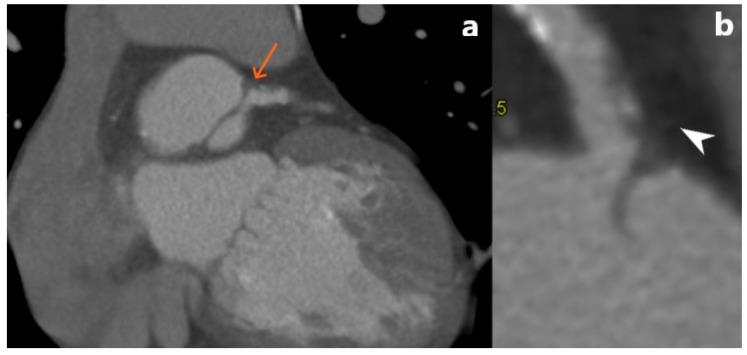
(a) Aortic root dissection spreading into the proximal portion of the LMA (arrow); (b) enlarged MD CT plane image showing the extension of the aortic dissection intimal flap within the proximal segment of the LMA (arrowhead). With noted pathologies on the aortic root as well as the LMA, the patient was referred to a vascular surgeon that indicated surgical treatment with elevated perioperative risk. However, the patient was not motivated, and therefore a conservative treatment with frequent follow-up was implemented.
